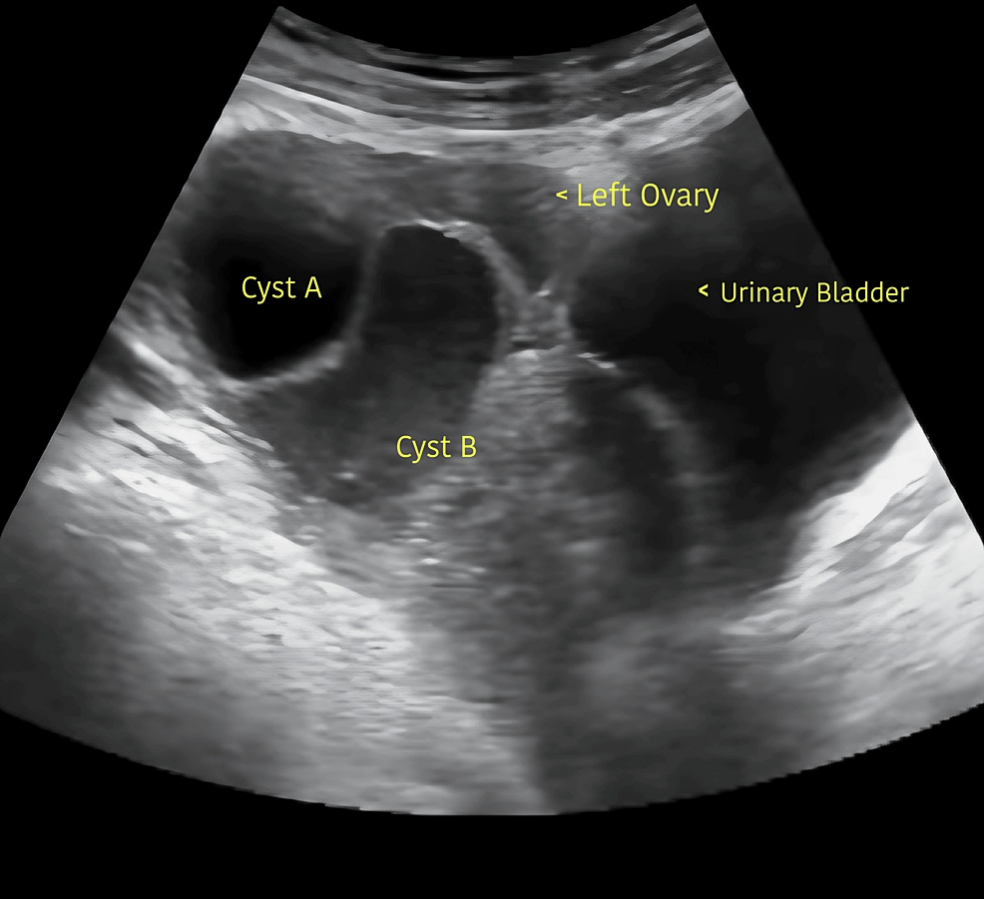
Ultrasound showing the left ovarian cyst A of size 3.2 x 4.3 x 3 cm and cyst B of size 10.2 x 9.3 x 9.6 cm
